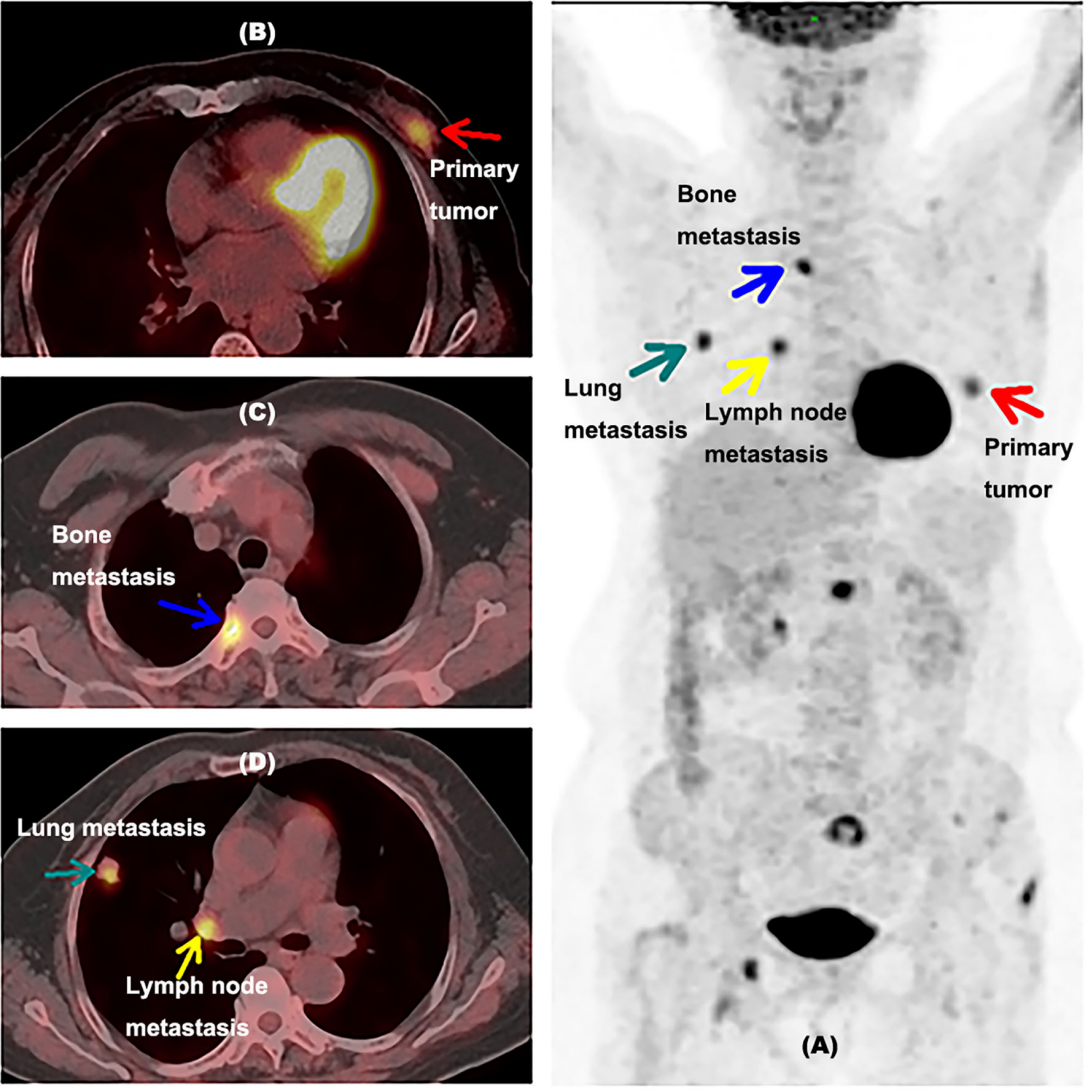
Example for a newly diagnosed breast cancer patient with unsuspected lung, hilar lymph node, and bone metastases detected by 18F-FDG PET/CT inspection. (A) A MIP picture of a 63-year-old newly diagnosed breast cancer woman detected by an ^18^F-FDG PET/CT inspection with the proportion of ER at 10%. The red arrow showed the primary tumor of this patient. The green arrow indicated lung metastasis in the right lung. The yellow arrow presented hilar lymph node metastasis. The blue arrow showed bone metastasis. (B) PET/CT fusion image of this woman with primary tumor. (C) PET/CT fusion image of this woman with lung metastasis and hilar lymph node metastasis. The green arrow indicated lung metastasis, and the yellow arrow presented hilar lymph node metastasis. (D) PET/CT fusion image of this woman with bone metastasis. ER, estrogen receptor; MIP, maximum intensity projection, with the proportion of ER lower than 25%.
